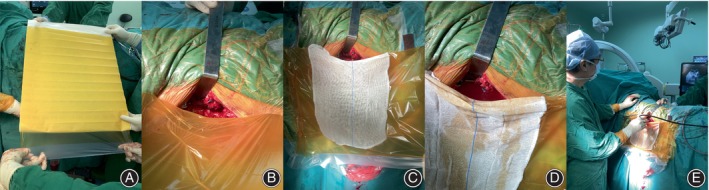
The manufacturing method of the bag containing normal saline for placing ultrasonic probe: another new antimicrobial incise drape was applied to cover the surgical area and adhered to the previously attached drape, with its upper edge ~3 cm higher than the incision, and a capsular bag (A, B) with an upward opening was made. The surgical area with no drape pasted was covered with sterile gauze to prevent the probe from adhering to it (C). The capsular bag and surgical field were filled with normal saline for acoustic coupling (D). A sterile 10‐MHz ultrasound probe was placed through the reserved window, and by scanning along the longitudinal axis and vertical to the body, sagittal and cross‐sectional images of the dural sac and neural elements were obtained (E).
